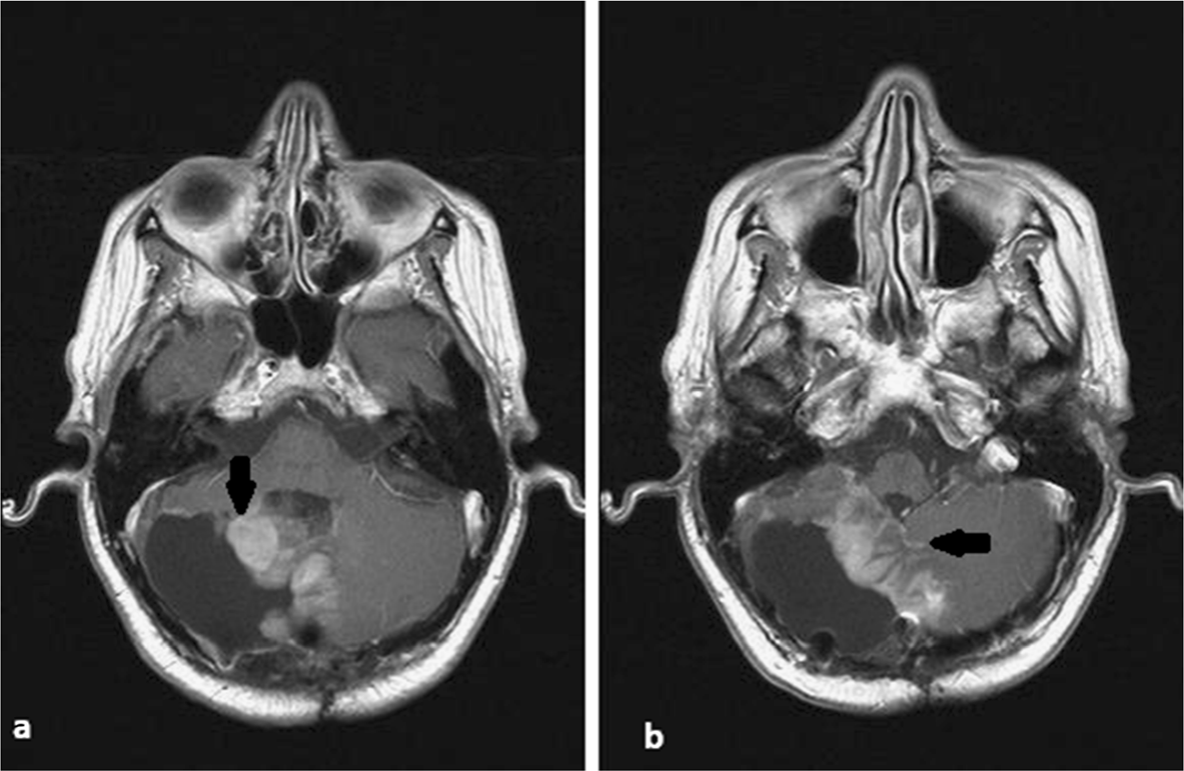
a and b: The magnetic resonance imaging before re-irradiation. Recurrence of disease is clearly evident (*arrows*).
